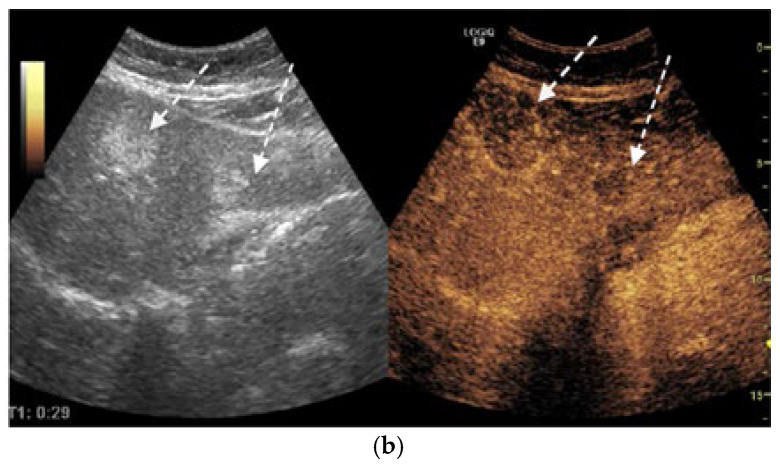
(a) Abdominal ultrasound B-mode image capturing two hyperechoic nodules in left liver lobe (segment IVa and III) with heterogeneous, irregular contours (white arrows). (b) Abdominal ultrasound in CEUS mode at the end of the arterial phase demonstrates earlier contrast washout of both nodules compared to the rest of the liver parenchyma (white arrows).
